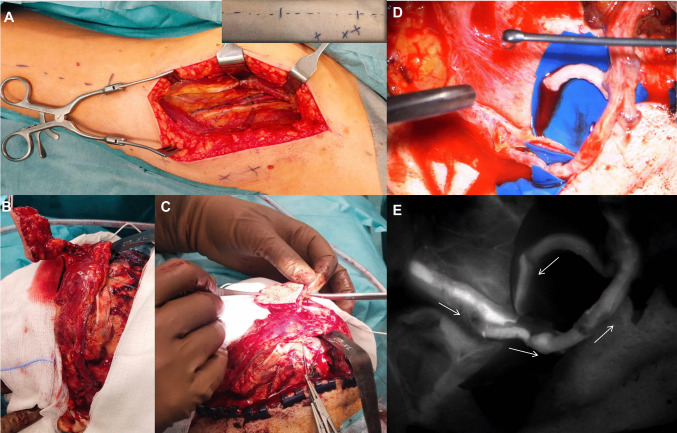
ALT perforator flap harvest and transfer. (A) Preoperative flap design on the lateral aspect of the left thigh based on Doppler/CTA-mapped perforators. (B) Intraoperative design of a perforator-based propeller flap according to actual perforator position. (C) Circumferential elevation of the flap, leaving an island paddle supplied by a single perforator pedicle. (D) Completed microvascular anastomoses (end-to-side artery, end-to-end vein). (E) ICG fluorescence angiography confirming good perfusion and patent anastomoses
